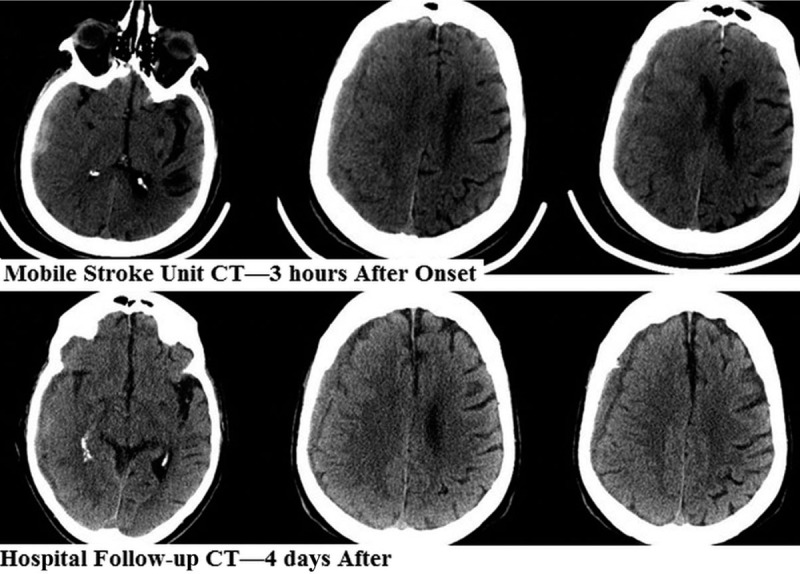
Computed tomography scan performed pre-Idarucizumab treatment in the mobile stroke unit demonstrating acute on chronic right subdural hemorrhage. Post-treatment scan in hospital demonstrates stability of the hemorrhage.
